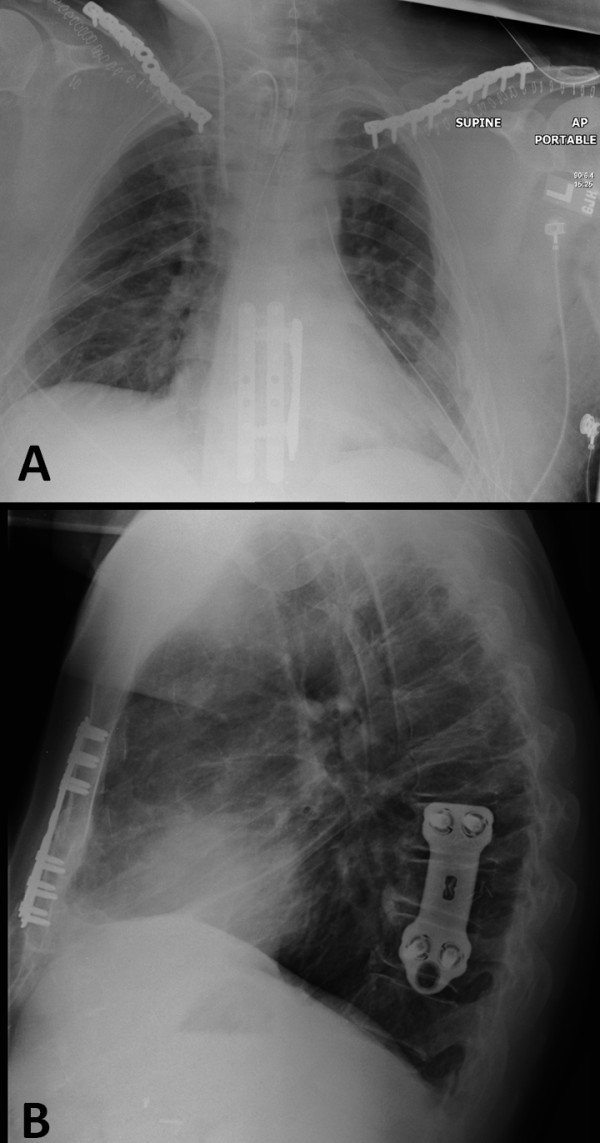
Radiographic documentation demonstrating the sternal fracture and T9 spine fixation in antero-posterior chest X-ray (A), and in the lateral plane at 6 months follow-up (B).
